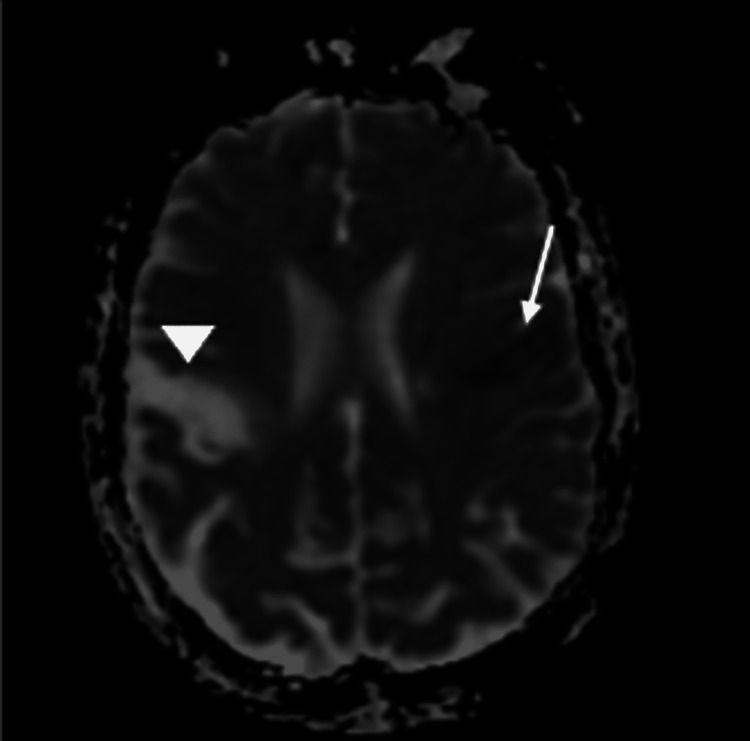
Apparent diffusion coefficient MRI of the brain showing decreased signal intensity in the region of the left frontal operculum (arrow), which confirms an acute left opercular stroke. Increased signal intensity in the region of the right frontal operculum (arrowhead) confirms a chronic infarct.
